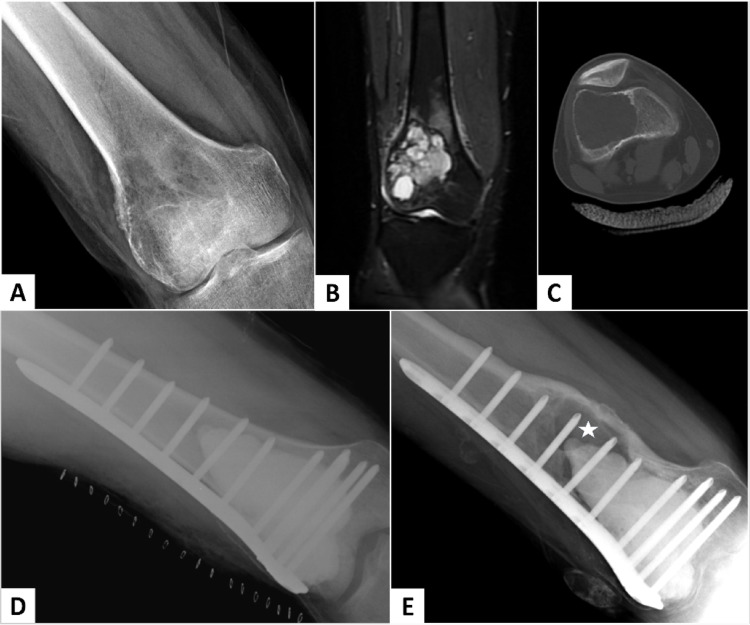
A 31-year-old patient with a giant cell tumor of the right distal femur. Preoperative lateral radiograph (A), coronal MRI (B), and axial CT image (C) show an expansile lytic lesion in the distal femur. Early postoperative radiograph after curettage, cementation, and plate fixation (D). Radiographic recurrence was observed around the cement mantle (asterisk) (E)
